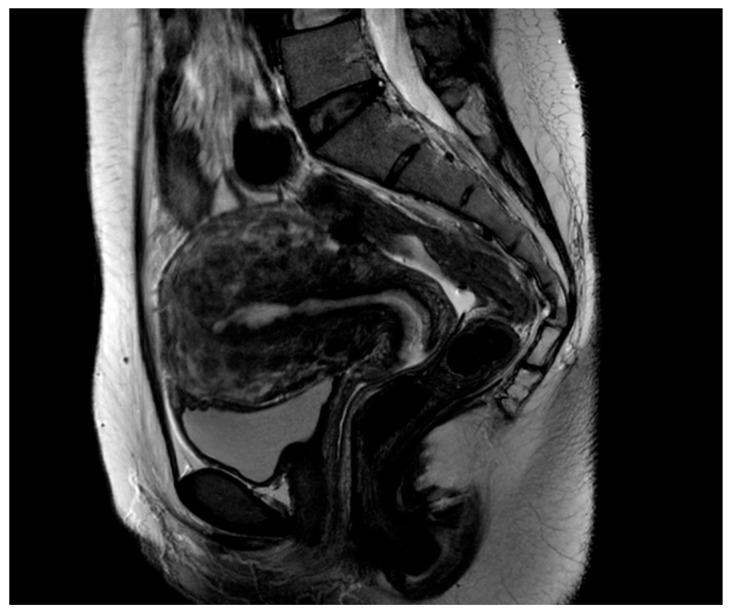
Sagittal T2-weighted magnetic resonance (MR) image shows multiple nodules with low-signal intensity in the whole uterus. An enlarged uterus shows diffuse innumerable submucosal, interstitial, and subserosal fibroids, with innumerable small fibroids blending and totally replacing the uterine parenchyma.
